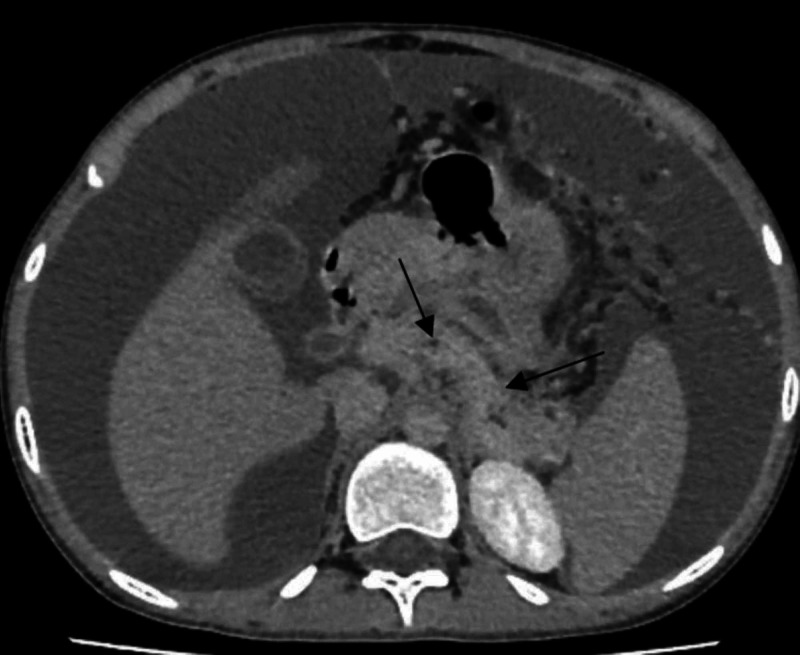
CT showing pancreatic enlargement with ill-defined border
